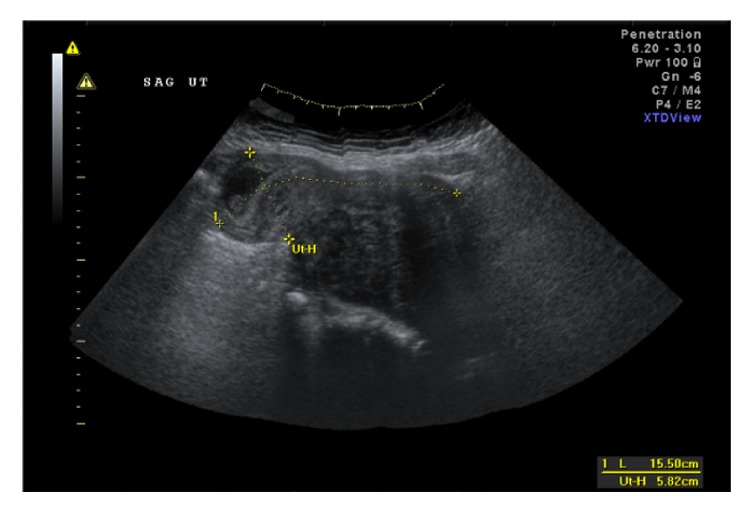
Ultrasound (US) image of fibroid uterus with intrauterine pregnancy.
